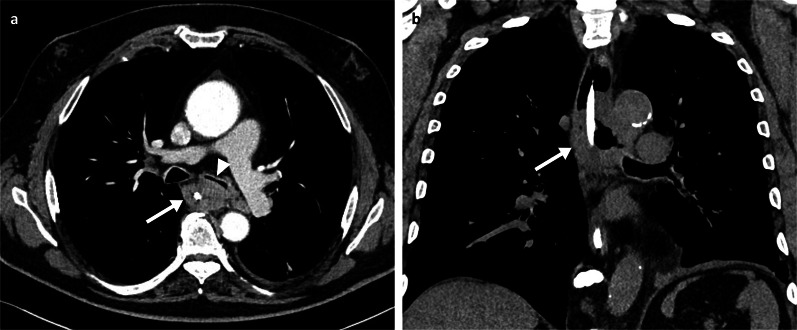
Arterial phase contrast-enhanced CT axial (a) and non-enhanced coronal (b) of a 58-year-old male patient demonstrating a large oesophageal metastatic melanoma mass (arrows) with luminal narrowing and compression of the left main bronchus (arrowhead). A nasogastric tube is in situ
